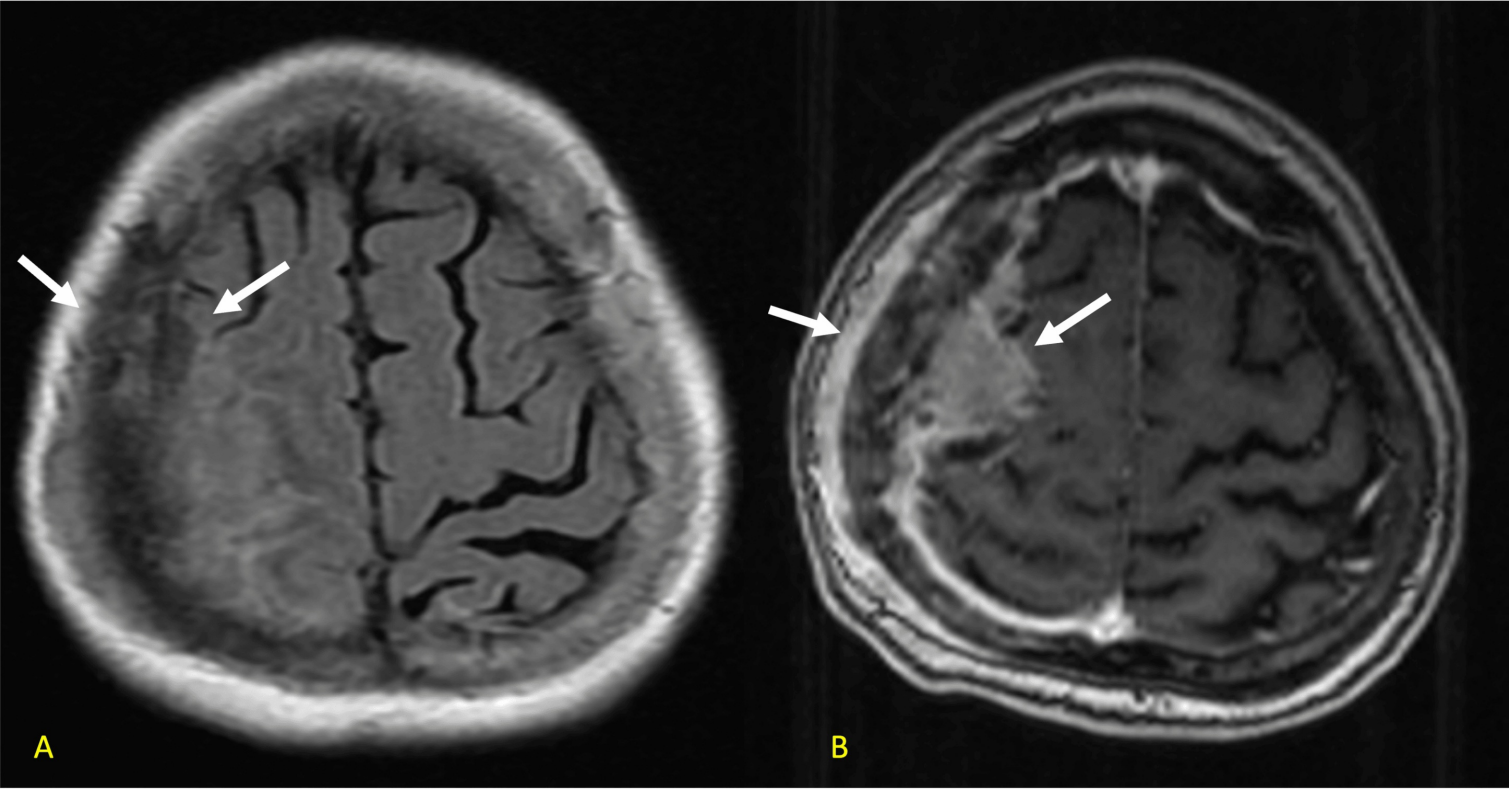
a. Axial T1 MRI brain images demonstrating metastatic infiltration of the right parietal bone demonstrating low signal marrow compared to the normal marrow fat high signal seen on the left. b. Contrast axial T1 showing mild diploic space enhancement with enhancing subgaleal and epidural masses along the right fronto-parietal convexity with adjacent frontal lobe compression.
